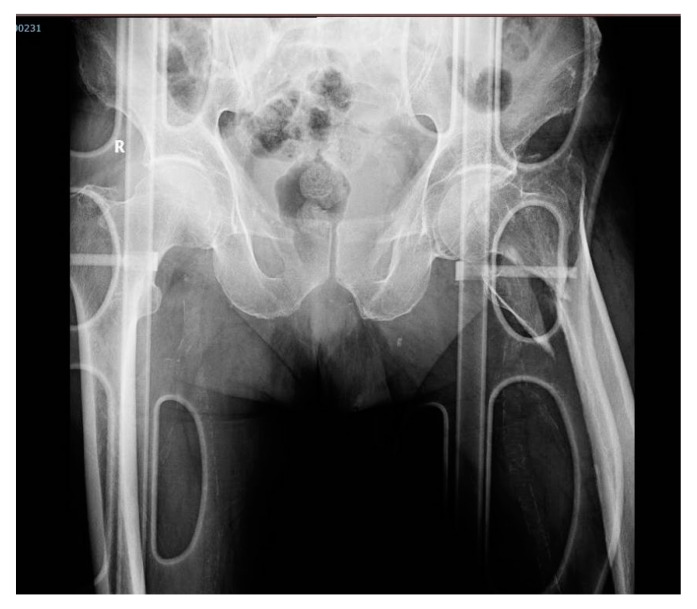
Pelvic X-ray upon arrival, in anteroposterior incidence. Description: Male subject on gurney. Right hip with minimal narrowing of the joint space. Left hip showing a left comminuted femur fracture in the upper ⅓ segment, with displaced bone fragments and minimum angulation.
